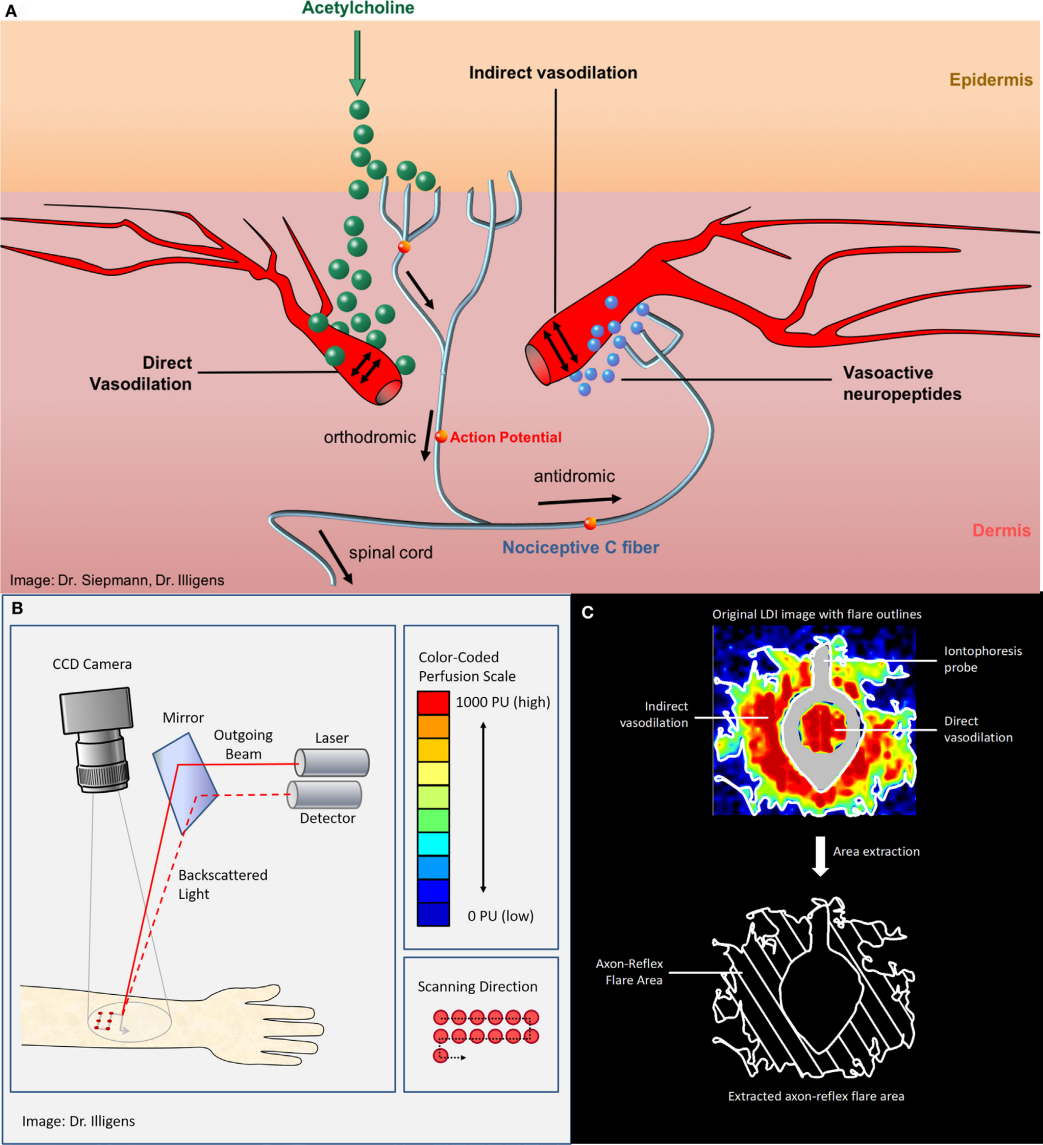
(A) The vasomotor axon reflex: iontophoresis of acetylcholine results in stimulation of peripheral epidermal nociceptive C-fibers and thereafter indirectly results in local vasodilatation. The topical stimulation depolarizes unmyelinated C-fibers in the skin and results in afferent action potentials that are conducted orthodromically toward the spinal cord. At branching points of the nerve, the impulse continues antidromically, ending at arterial dermal vessels adjacent to the initial stimulation point. There, the axon reflex induces the release of vasoactive substances, such as substance P and calcitonin gene-related peptide, from nerve terminals and leads to vasodilation. (B) Laser Doppler imager setup and principle of measurement. (C) The upper panel shows the color-coded laser Doppler imaging perfusion data with iontophoresis probe framing direct vasodilation induced by direct stimulation as well as axon reflex-mediated enhancement of blood flow (“flare”) in an adjacent skin area surrounding the probe (“indirect flare”). The lower panel shows the extracted axon reflex flare, which provides a measure of functional integrity of those nociceptive C-fibers that mediate the vasomotor axon reflex upon activation through acetylcholine.
